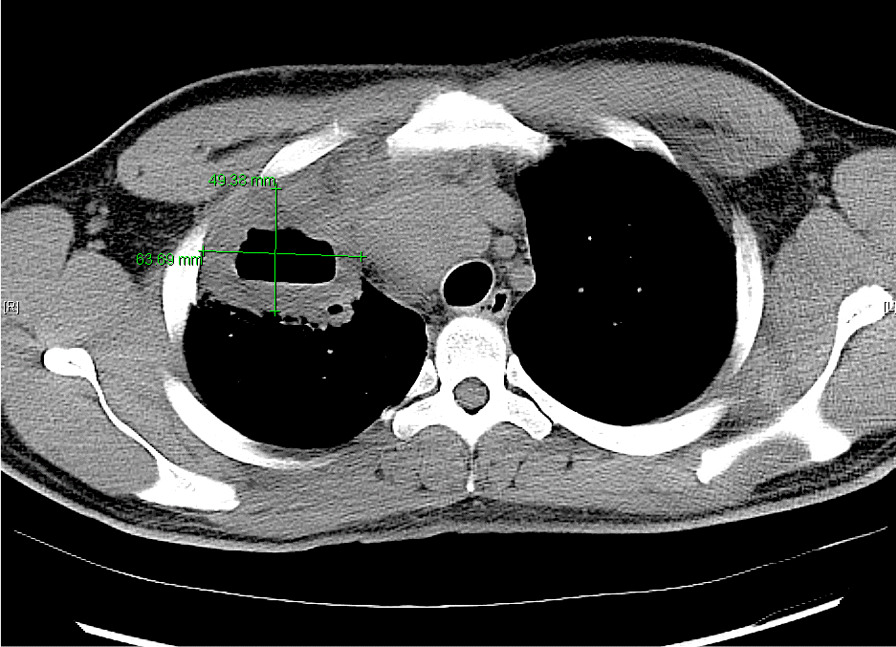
Initial CT chest (axial image). Right upper lobe cavitary lesion
